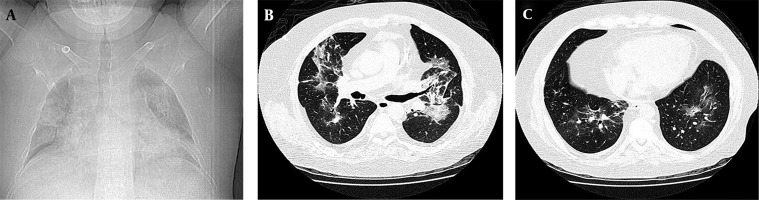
Chest X-ray and CT Section Images Results A. CXR of diffuse bilateral perihilar opacity with extension to periphery of upper and lower lobes; B, C. CT section images, bilateral perihilar alveolar ground glass opacity with extension to periphery of the upper and lower lobes.
